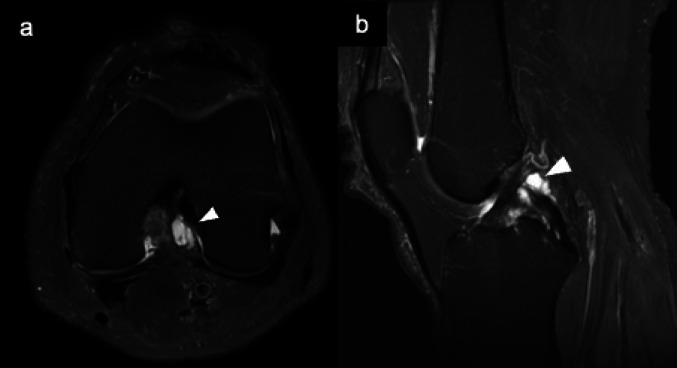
Intra-articular ganglion. Axial (a) and sagittal (b) fat-suppressed T2-weighted MR images demonstrate a septated, high signal intensity mass (arrows) located on either side of the posterior cruciate ligament (PCL)
